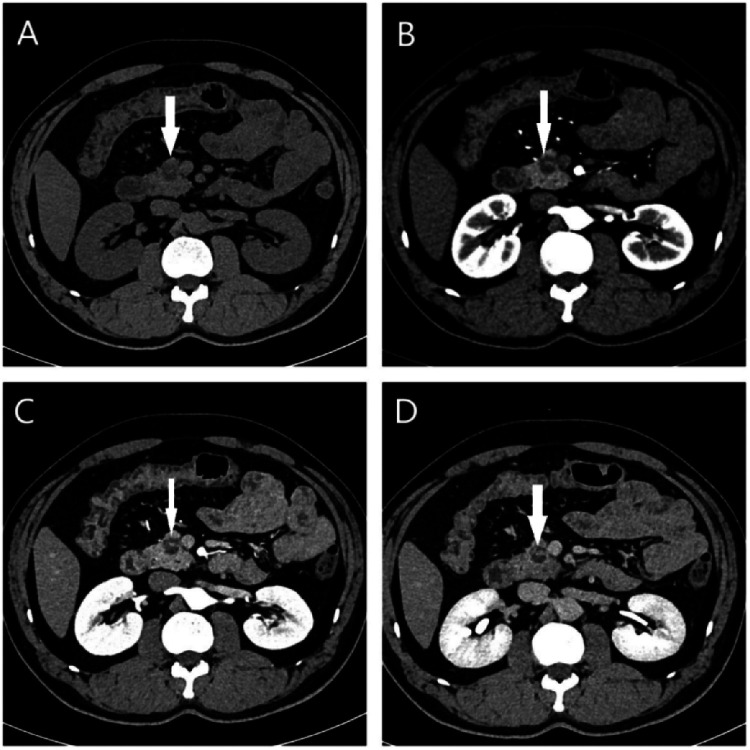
(A) On the plain CT scan, a small lesion with heterogeneous density and indistinct borders located in the pancreatic head (white arrow) were observed. Contrast-enhanced axial CT images showed the mass (white arrow) with heterogeneous enhancement in the (B) arterial, (C) venous and (D) delay phase.
